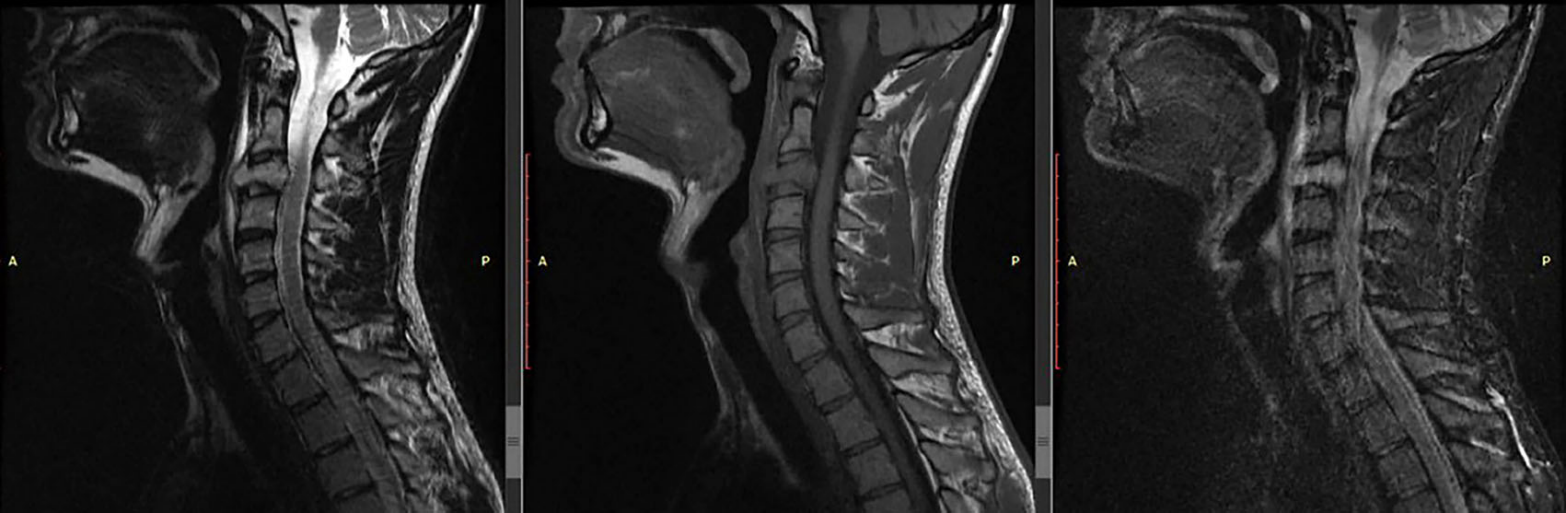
Pre-operative MRI showed C3 vertebral body collapse with epidural and prevertebral soft tissue collection causing cord.
